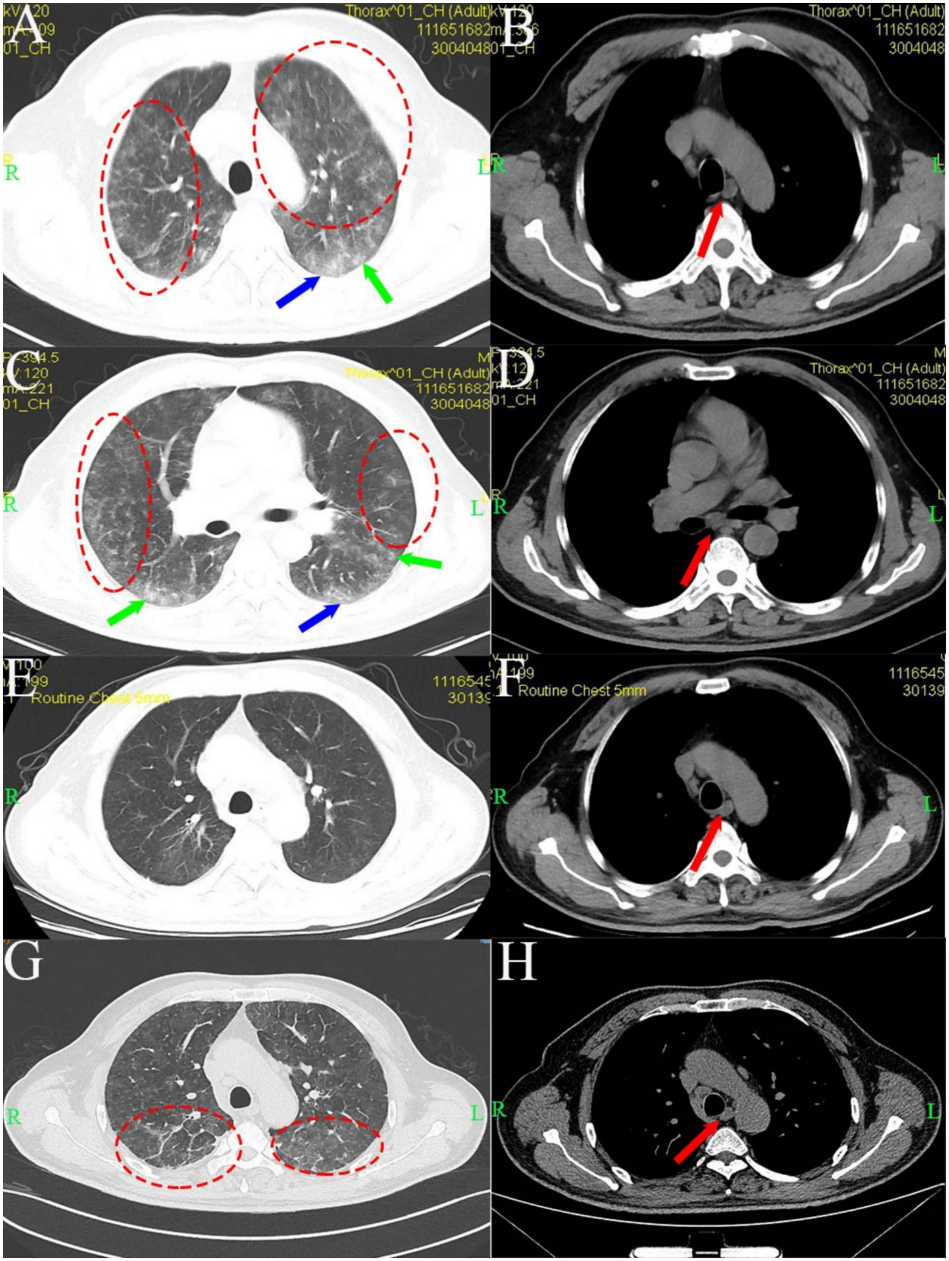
Chest CT imaging showing treatment response and subsequent recurrence. Initial CT (A–D) demonstrates diffuse bilateral ground-glass opacities, nodules, and peripheral reticulations (most prominent in the lower lungs), accompanied by mediastinal lymphadenopathy. Follow-up CT after a 10-day course of methylprednisolone and antimicrobial therapy shows marked resolution of the pulmonary lesions (E,F). A subsequent HRCT confirms recurrence, with extensive ground-glass/reticular opacities and persistent mediastinal lymphadenopathy (G,H). Red dashed circles indicate ground-glass opacities; green arrows indicate nodular opacities; blue arrows indicate reticular opacities; red arrows indicate lymphadenopathy.
